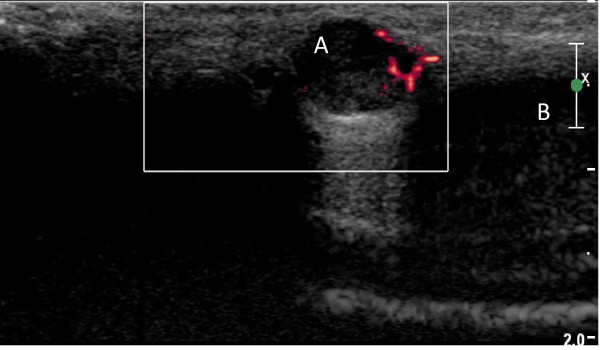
Ultrasound scan image of the penis. (A) Ultrasound picture shows the epithelioid hemangioma lesion on the dorsum of the penis. Inherent vascularity is visible within the lesion in red color. (B) Both corpora cavernosa can be seen.
